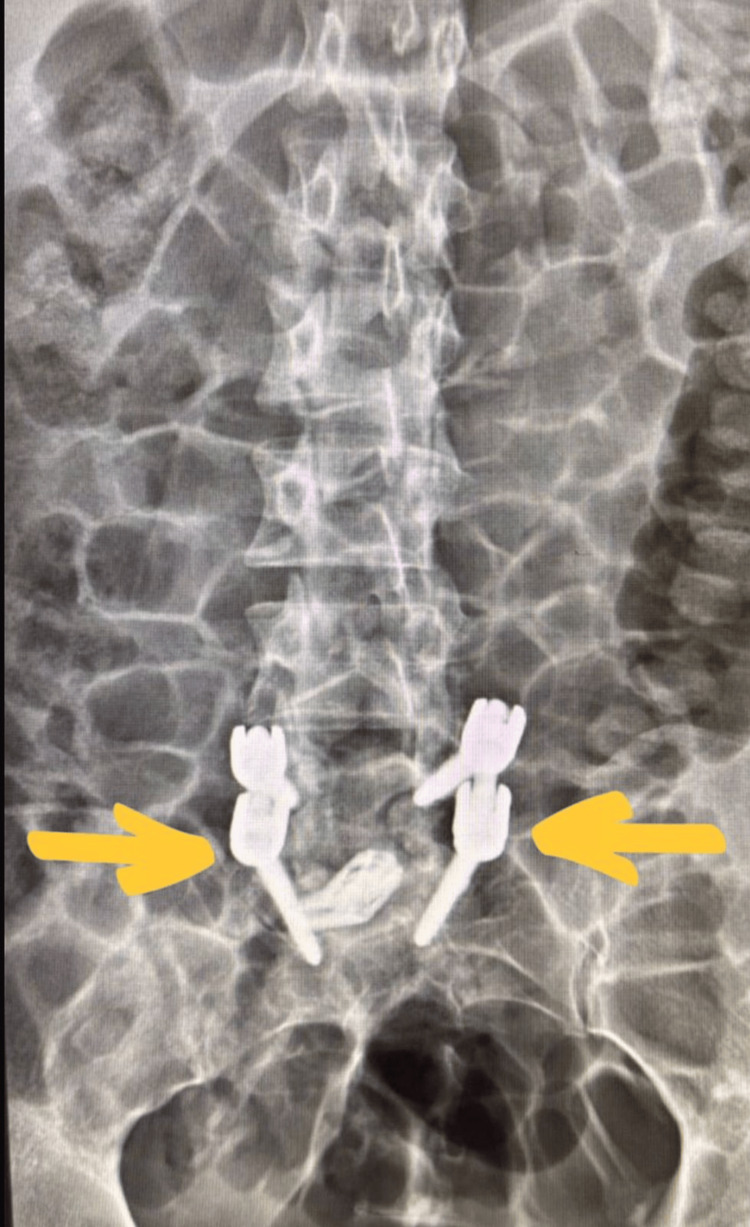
Postoperative anteroposterior X-ray showing pedicle screw fixation at L5–S1 following decompression and fusion.
